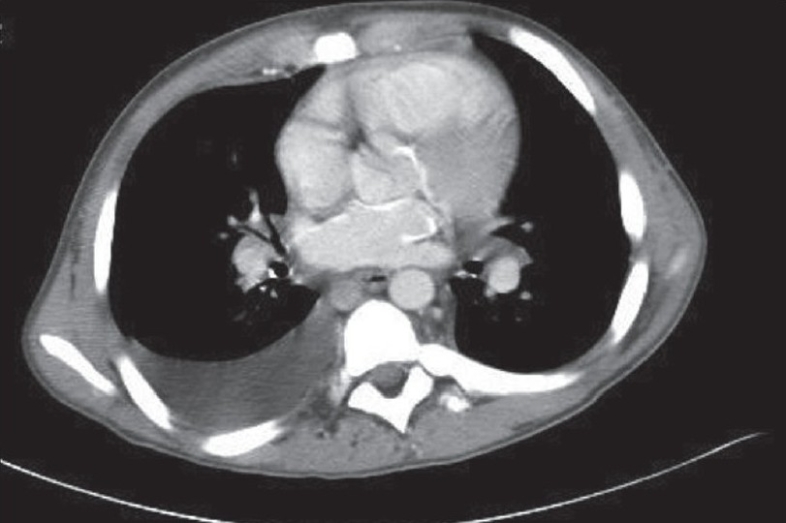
Chest CT scan showing cardiac calcification involving left atrium aortic root, proximal coronaries and mitral valve ring
